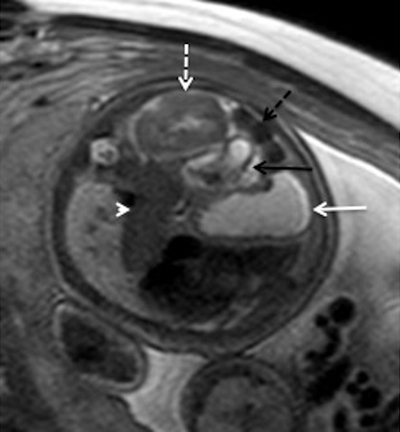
Left diaphragmatic hernia content. Axial T2-weighted sequence on a 33 week-old foetus with left diaphragmatic hernia. The content of the hernia is well characterized showing the stomach (white arrow), the spleen (arrow head), the kidney (dotted arrow), the small bowel (black arrow) and the colon filed with meconium (dotted black arrow).
